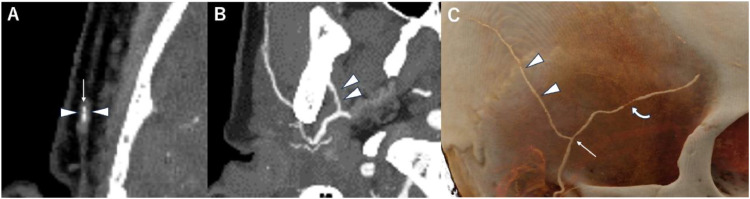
CT 2 months after initiation of steroid treatment. (A) CT angiography at the bifurcation of the frontal and parietal branches of the right superficial temporal artery shows a slight improvement of wall thickening (arrowheads) with residual luminal stenosis (arrow). (B) A thin-slab maximum intensity projection image at the maxillary artery demonstrates luminal stenosis (arrowheads). (C) The cinematic rendering image demonstrates an improvement in stenosis at the bifurcation (arrow) and the parietal branch of the right STA (arrowheads). Additionally, it reveals the emergence of stenosis at the frontal branch (curved arrow).
